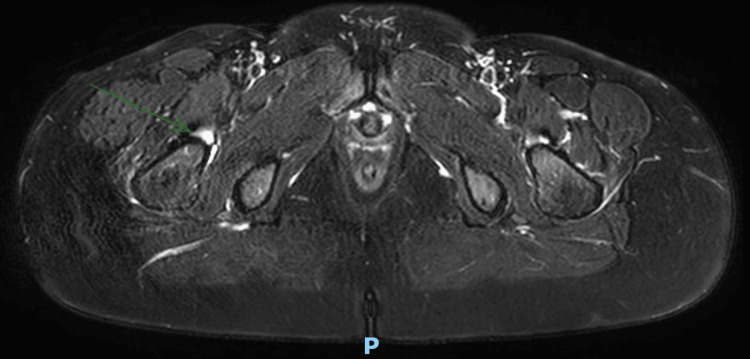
T2-Weighted MRI: Axial cut Gluteus medius enthesopathy (arrow).
